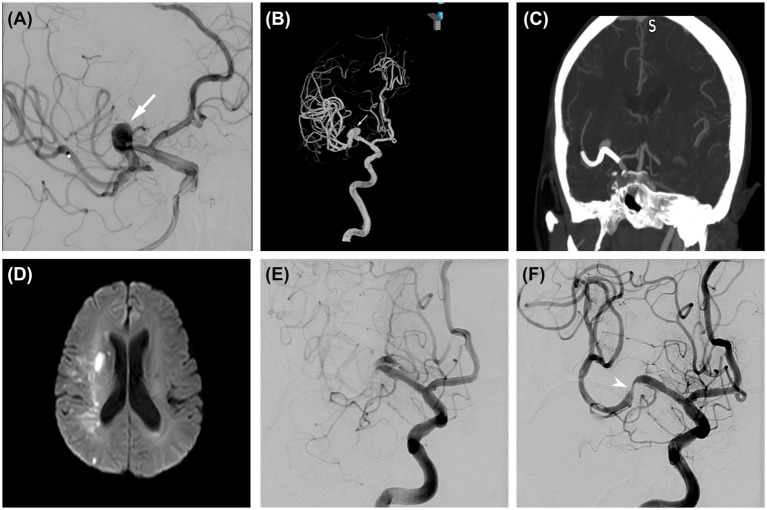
(A) Preoperative angiography showing a saccular aneurysm (arrow) in the M1 segment of the right middle cerebral artery. (B) 3D reconstruction of DSA showing the saccular aneurysm (arrow). (C) Postoperative computed tomography angiography showing that the flow diverter device completely covered the neck of the aneurysm and multiple branches. (D) On postoperative day 15, the patient developed sudden left limb weakness. DWI indicated an acute infarction in the right basal ganglia region. (E) Cerebral angiography showed slow blood flow through the stent and delayed opacification of distal vessels, suggesting in-stent thrombosis. (F) After thrombus aspiration and stent remodeling, blood flow through the stent improved, and the opacification time of distal vessels was reduced (arrow).
